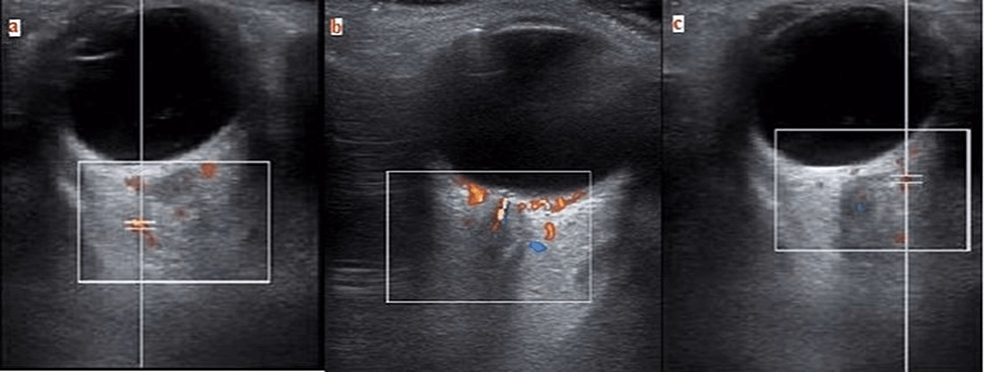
Measuring technique of ophthalmic artery (a), central retinal artery (b), and posterior ciliary artery (c) with orbital Doppler
